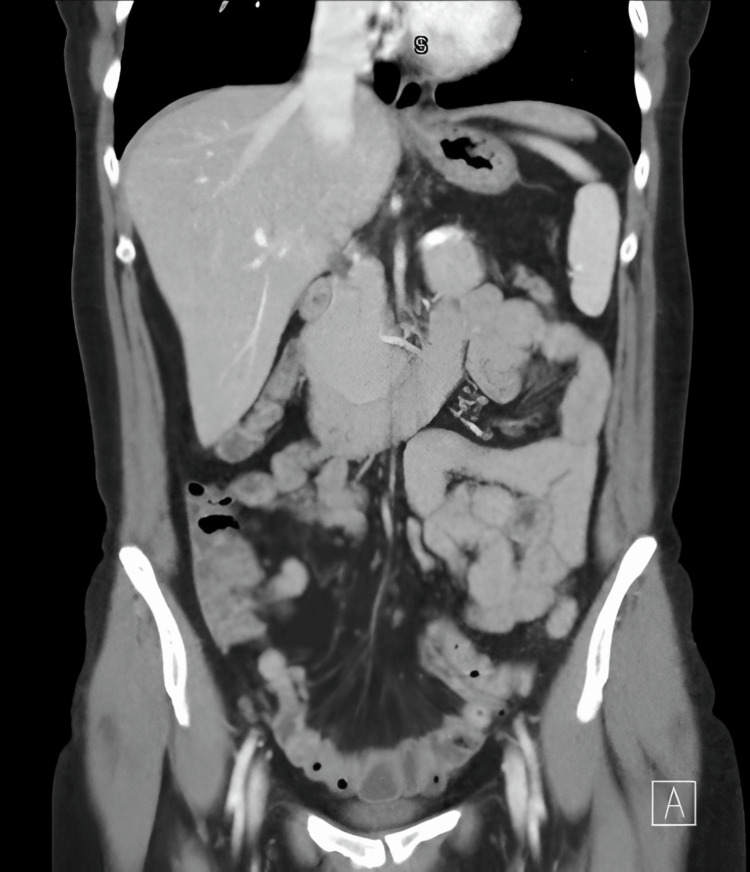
Coronal CT image of the abdomen and pelvis obtained without IV contrast CT: computed tomography, IV: intravenous
